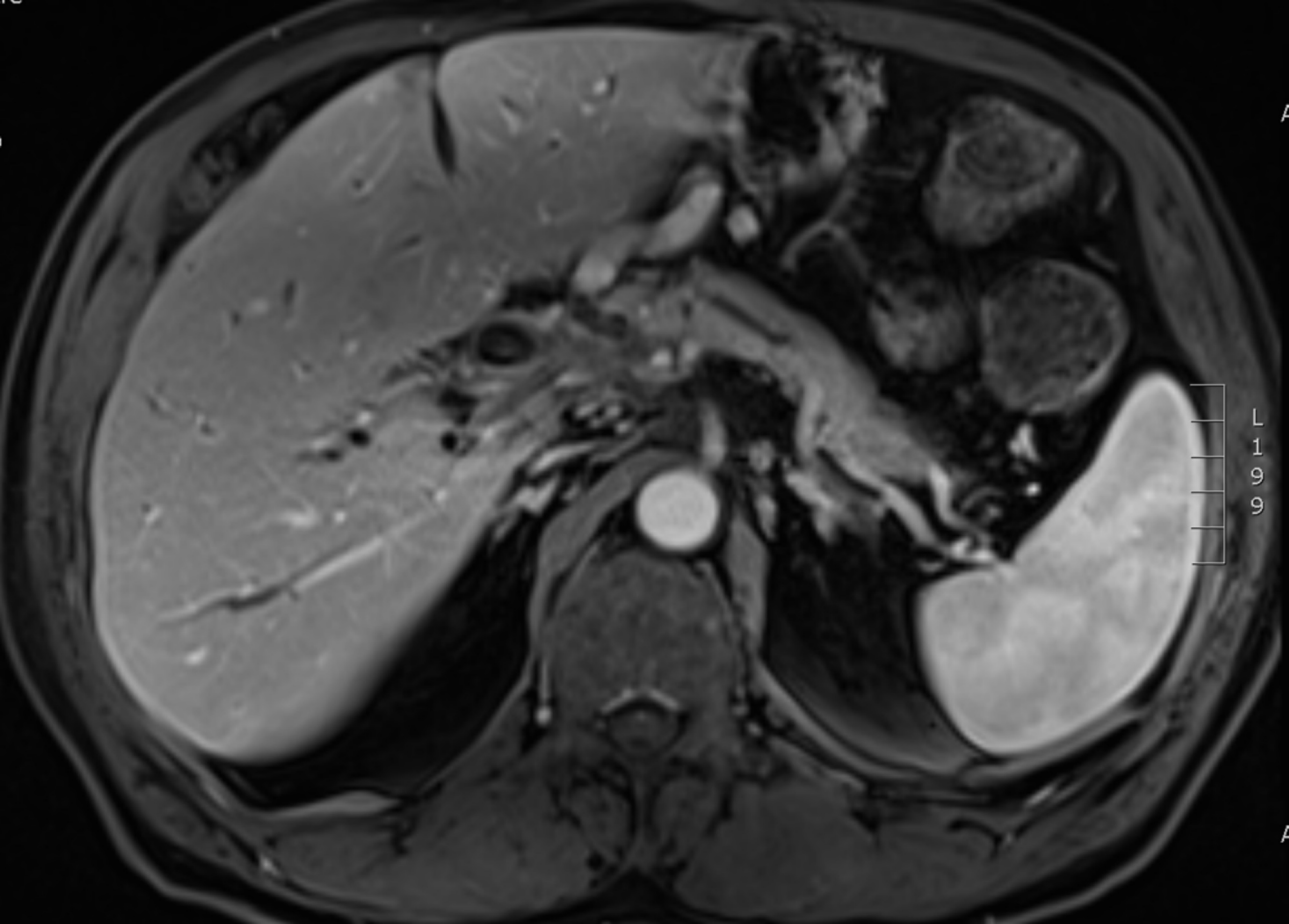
Venous phase MRI liver (VIBE sequence) obtained four months post-SBRT to the NSCLC demonstrating the complete resolution of the hepatic metastasis.
